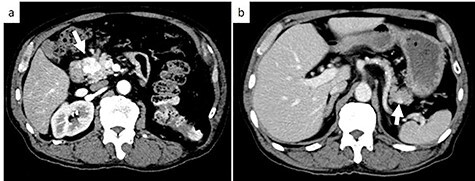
Axial contrast-enhanced CT images; (a) CT showing a 25-mm well-defined hypervascular heterogeneous lesion in the pancreas head; (b) CT showing a 9-mm well-defined hypervascular heterogeneous lesion in the pancreas tail. White arrows indicate the tumor in the pancreas head and tail.
